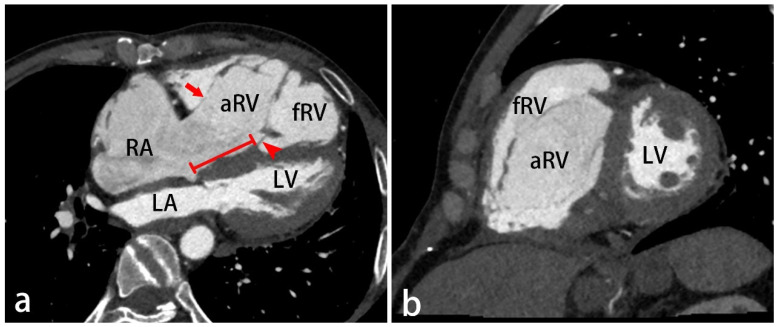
A 17-year-old male with Ebstein anomaly. Four-chamber view (a) and two-chamber view (b) of CT angiography shows inferior displacement of the septal leaflets of the tricuspid valve ((a), red arrowhead), resulting in an atrialized right ventricle and reduction in the size of the functional right ventricle. The degree of functional tricuspid valve annular displacement can be quantified by measuring the distance between the hinge points of the septal leaflet and the anterior leaflet of the mitral valve ((a), red line). The antero-superior leaflet has multiple attachments to the right ventricle wall, showing “sail-like” appearance ((a), red arrow). LV, left ventricle; LA, left atrium; RA, right atrium; aRV, atrialized right ventricle; fRV, functional right ventricle.
